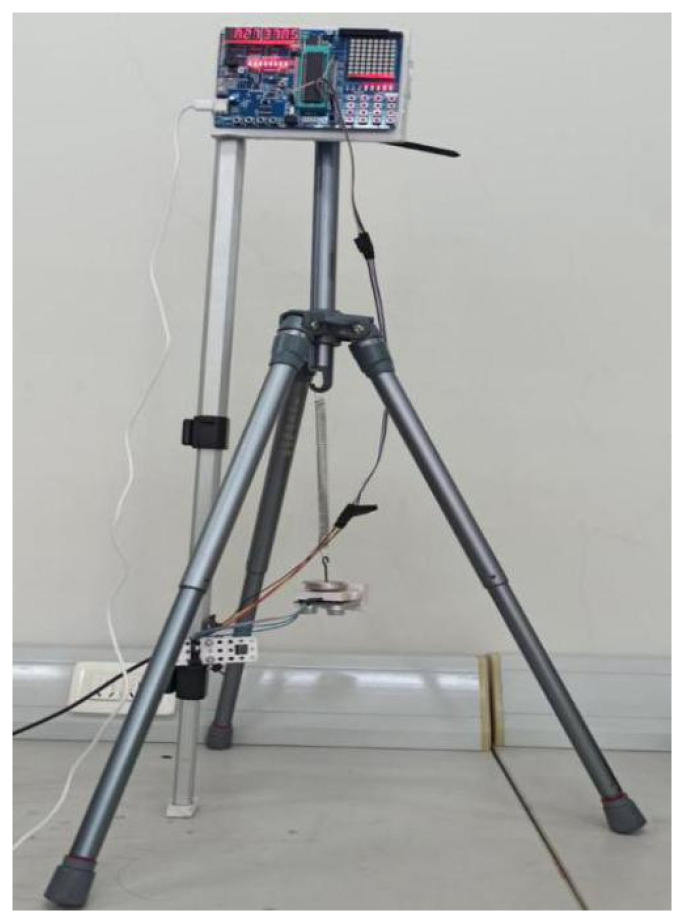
The stiffness factor *k* of the spring is measured by our ultrasonic testing instrument.
